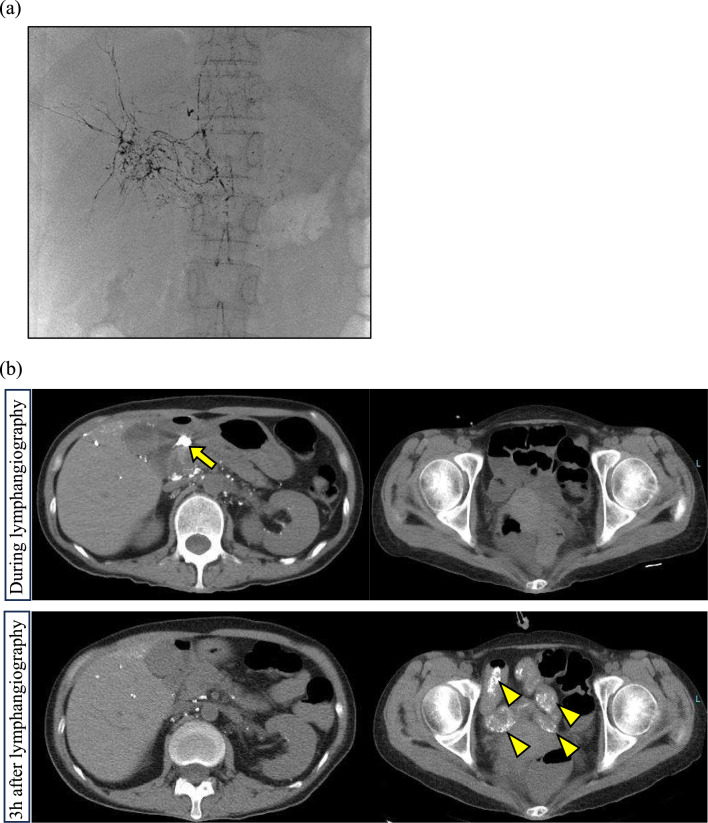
(a) Percutaneous transhepatic lymphangiography was performed under ultrasound guidance. A 25G catheter needle was inserted into the Glisson's capsule proximal to the anterior segment of the right lobe of the liver. Contrast medium was then injected, and lymphatic vessels was observed. It showed no obvious cisterna chyle. (b) CT images taken during lymphangiography (upper) and three hours later (lower) are shown. During lymphangiography, lymphatic leakage from the posterior wall of the duodenum was observed (arrow). However, after three hours, lipiodol was no longer pooled in the duodenal wall and had flowed into the small intestine (arrowhead)
